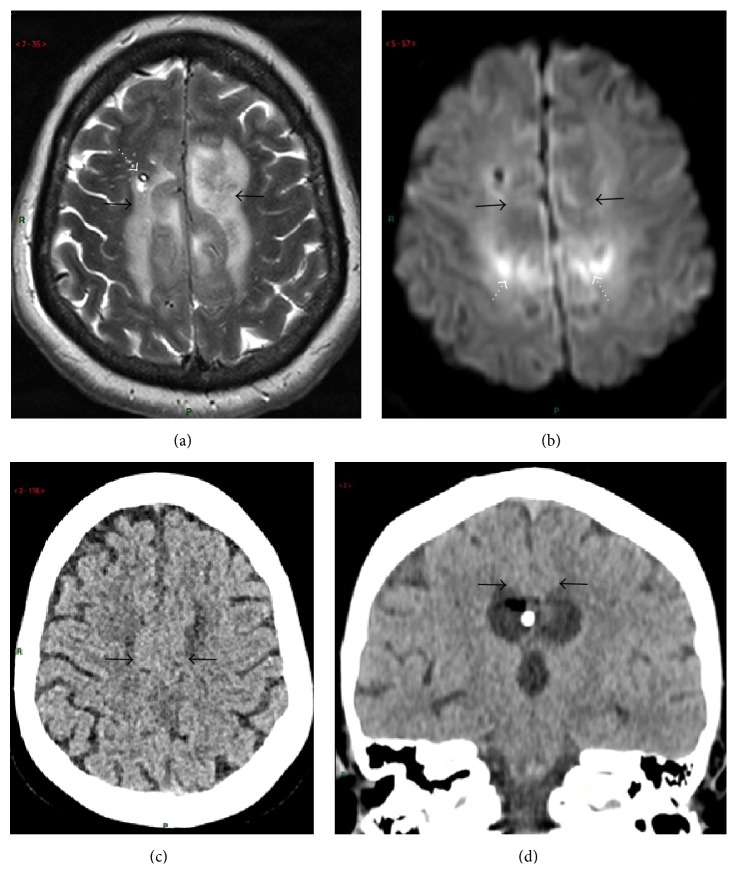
MRI work-up (a-b) at acute phase and delayed (4 months) CT scanner follow-up imaging (c-d) of patient 2. (a) T2-weighted fast-spin echo (FSE) axial transverse view through cingulate gyri demonstrating oedema-related strong hypersignal intensity within them on both sides. Ventricular draining catheter is seen on right frontal area (dotted white arrow). (b) Axial transverse diffusion-weighted (*b* factor = 1000 s/mm^2^) view in similar slice location failed to reveal hypersignal intensity within the same cingular areas (black arrows), thereby excluding ischaemic cytotoxic oedema. Only false positive artifacts due to adjacent hematoma were seen (arrows). (c-d) CT scanner axial transverse (c) and coronal reformatted (d) images from helical acquisition demonstrating integrity of the cingulate gyri (arrows).
